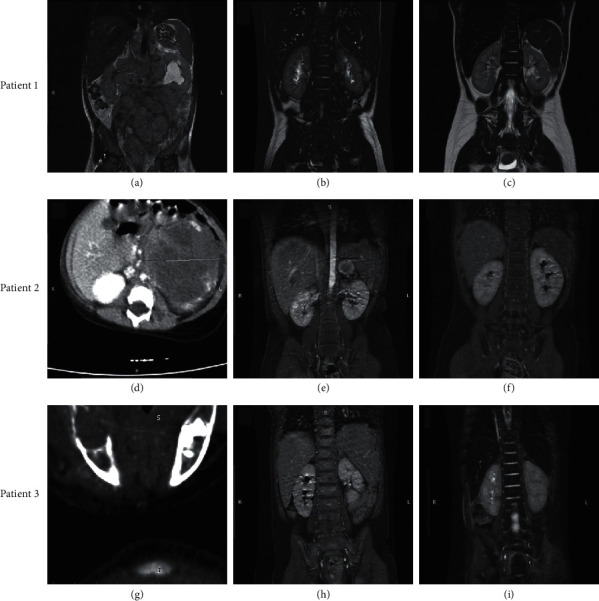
Abdominal MRI scans for Patient 1 (a–c), Patient 2 (d–f), and Patient 3 (g–i). MRI scans for Patient 1: (a) at diagnosis, (b) following induction therapy with eight cycles of COJEC, a cycle of adjuvant dinutuximab beta, and two cycles of adjuvant TVD, and (c) at the end of maintenance therapy with dinutuximab beta. MRI scans for Patient 2: (d) at diagnosis, (e) following induction therapy with eight cycles of COJEC, and (f) following three cycles of dinutuximab beta maintenance therapy. MRI scans for Patient 3: (g) at diagnosis, (h) following induction therapy with seven cycles of COJEC, and (i) following three cycles of second-line immunochemotherapy with irinotecan/temozolomide and dinutuximab beta and surgery of the primary abdominal tumor.
